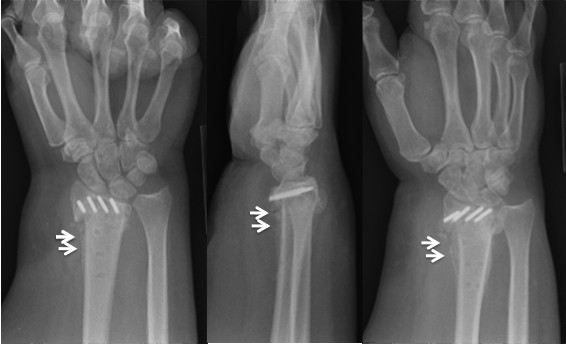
Twelve months after the removal of the plate, patient continued to have symptoms. Patient refused to have CT scan of her wrist as she had severe claustrophobia. Radiographic view obtained at the time showing bridging callus (arrows), however the diagnosis of nonunion was still not excluded before the surgery.
